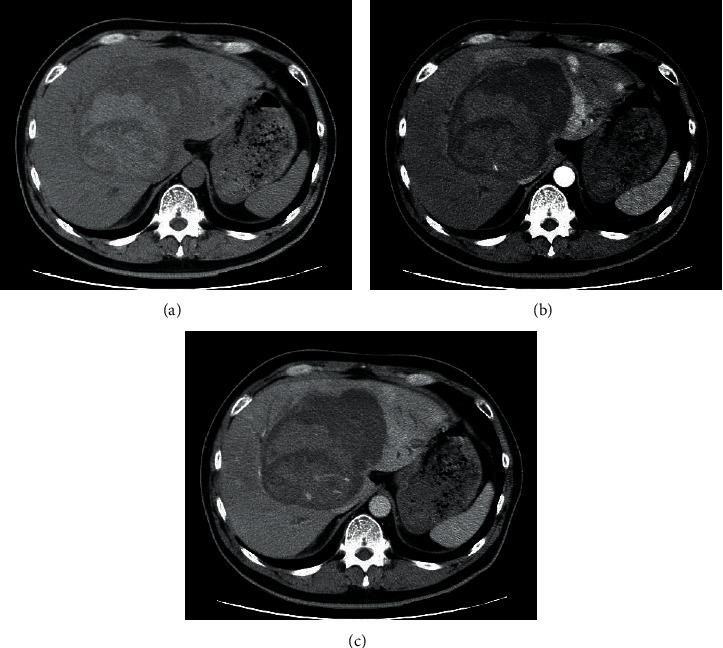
(a) Plain abdominal CT showing an irregular high-density area in the center of the liver and bleeding is suspected. Tumor detection is difficult. (b) Contrast-enhanced CT (arterial phase) revealing multiple tumors with a contrast effect in the left lobe of the liver (arrow). The patient was diagnosed with tumor-associated hemorrhage. (c) Abdominal CT (late phase) disclosing that the tumor exhibits the same contrast effect as normal liver tissue.
